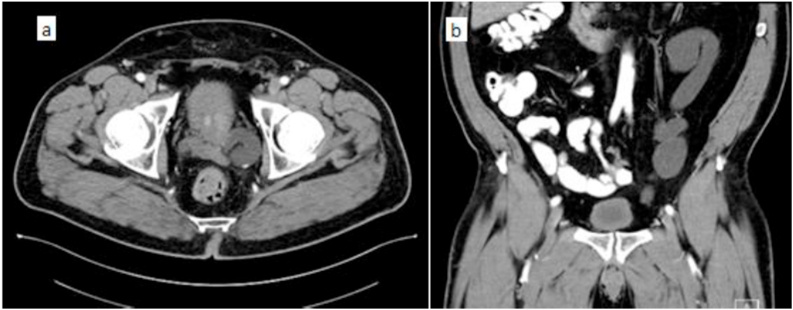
Preoperative CT scan: (a) axial image showing dilated left ectopic ureter twisting behind the prostate and (b) coronal image showing left upper pole hydroureteronephrosis.
